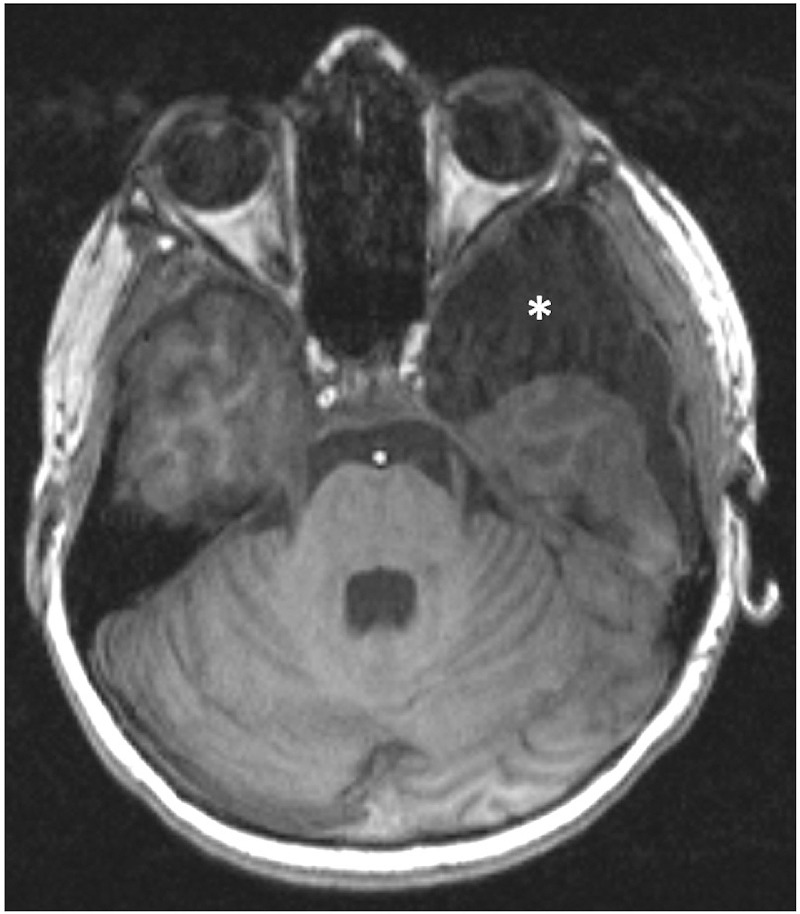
Representative example of an unenhanced 3D MPRAGE brain image with motion artifacts of the lens and vitreous body owing to eye movements, which was rated as insufficient by both readers. MRI also demonstrated a left temporal lobe arachnoid cyst (*).
